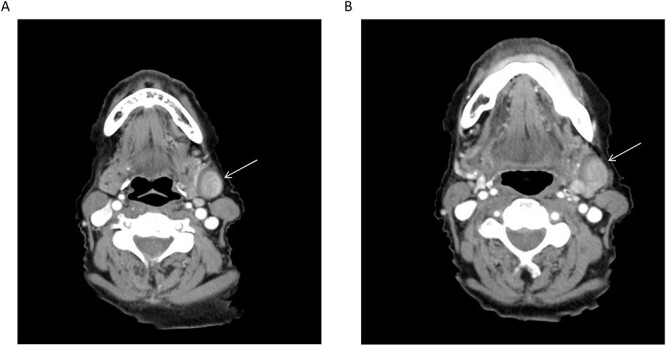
(A, B) Axial CT cuts of the neck with contrast revealing the lesion in the left submandibular neck (arrow); retrospective review demonstrates communication of the lesion with the adjacent left external jugular vein.
